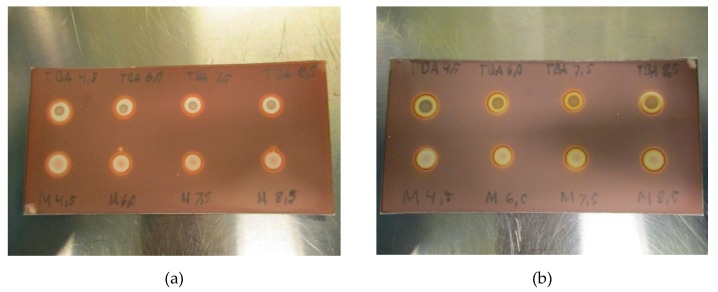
(a): TBA and matrix spots pH-range 4.5–8–5 for [^18^F]PSMA-1007 without antioxidant and (b): TBA and matrix spots pH-range 4.5–8–5 for [^18^F]PSMA-1007 with antioxidant.
